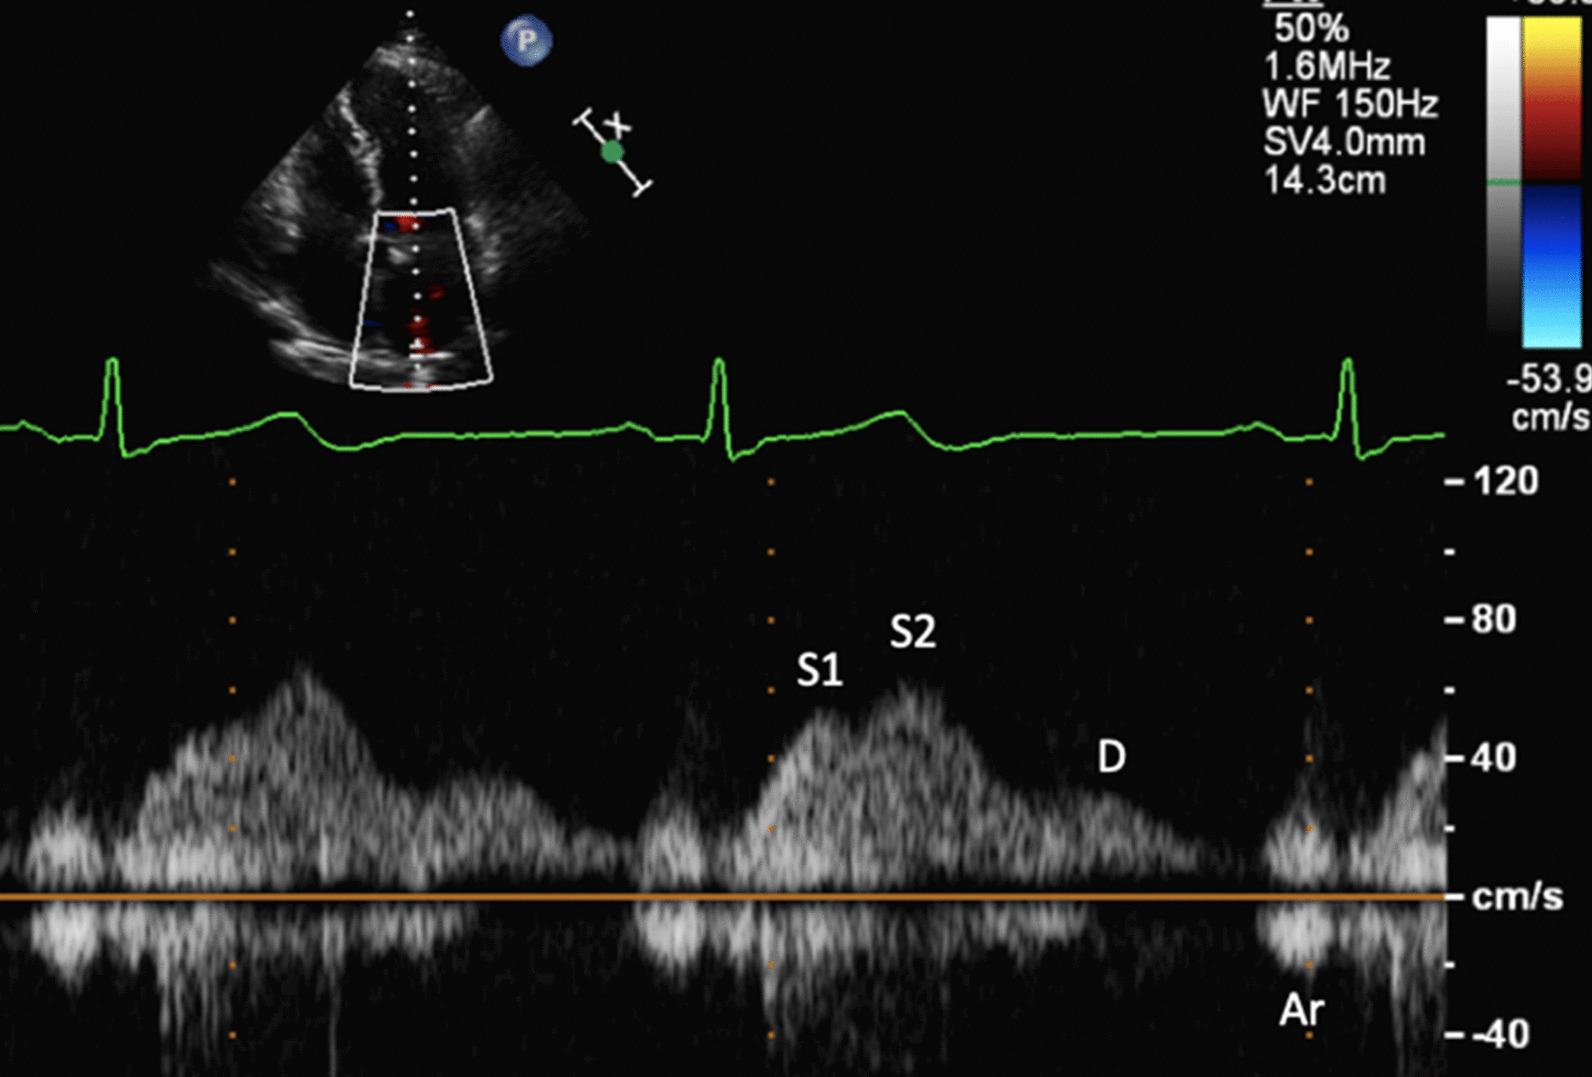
Pulsed Wave Doppler spectral display of pulmonary vein flow. S1 and S2 waves reflect left atrial filling during LV systole (LA reservoir phase). The D wave reflects pulmonary vein flow during early ventricular diastole (LA conduit phase). The Ar wave reflects flow reversal within the pulmonary veins secondary to atrial contraction (LA pump phase)
